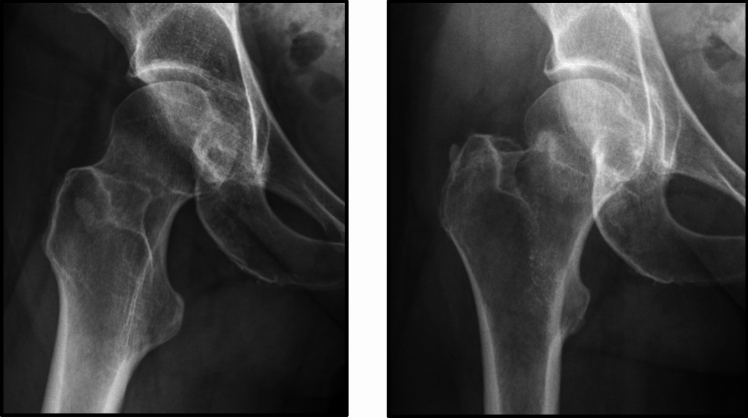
Radiographs of a valgus impacted femoral neck fracture
